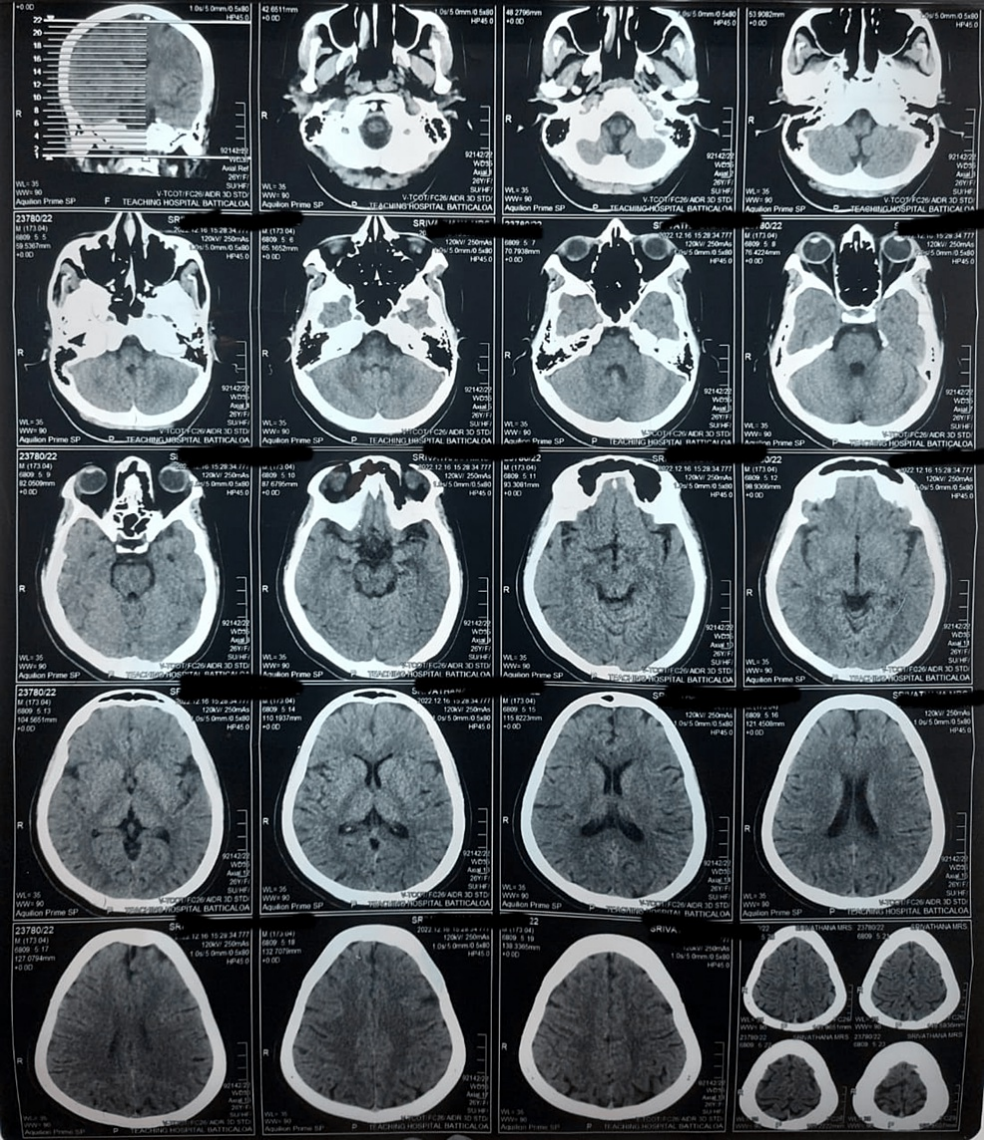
Non-contrast CT (NCCT) Brain of the patient- Normal NCCT Brain
